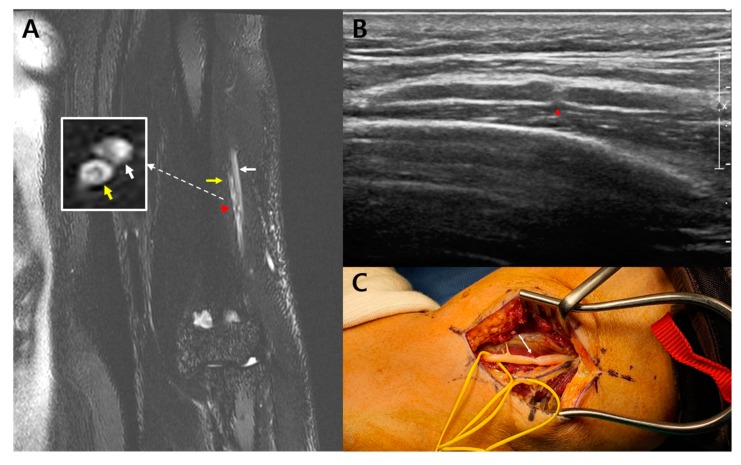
Case 1: (A) High-resolution magnetic resonance neurography reveals a focal constriction (red arrowhead) of the left posterior interosseous nerve (PIN) (yellow arrows) within the epineurium of the left radial nerve 8.9 cm proximal to the lateral epicondyle. The left superficial radial nerve (white arrows) is swollen. Further, the bull’s eye sign (yellow arrow in white square) is observed juxta-proximal to the constriction site of the left PIN; (B) Longitudinal ultrasound reveals constriction (red arrowhead) of the left radial nerve; (C) Intraoperative finding confirms constriction (write arrow) of the left radial nerve.
